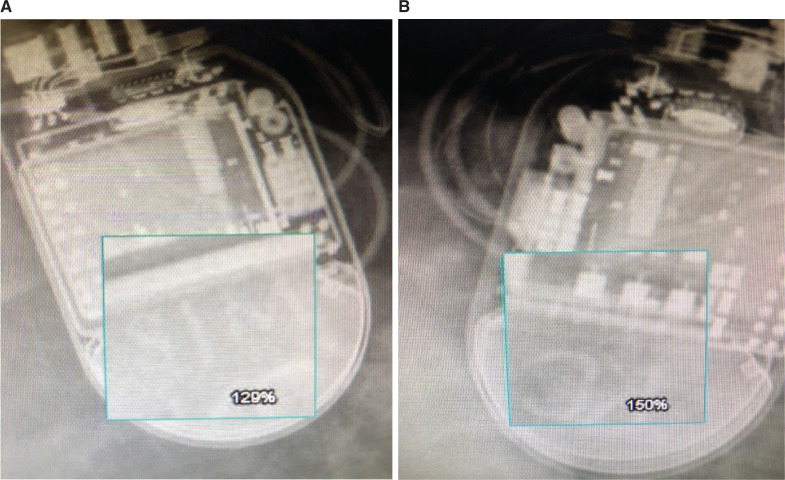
A: The X-ray logo of an Abbott (St. Jude Medical) defibrillator is visible although faint in front-to-back orientation. B: The X-ray logo of a similar Abbott (St. Jude Medical) defibrillator model is not visible in a tilted back-to-front orientation.
